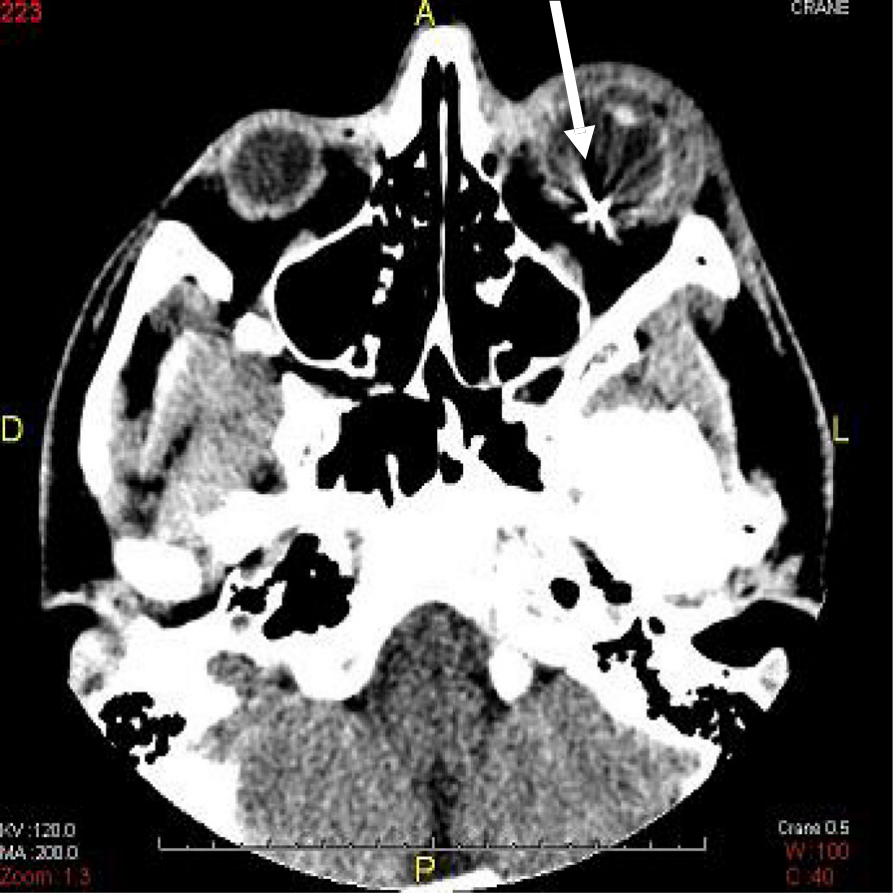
Orbital computed tomography demonstrates a metallic intraocular foreign body with edema of the left eye
